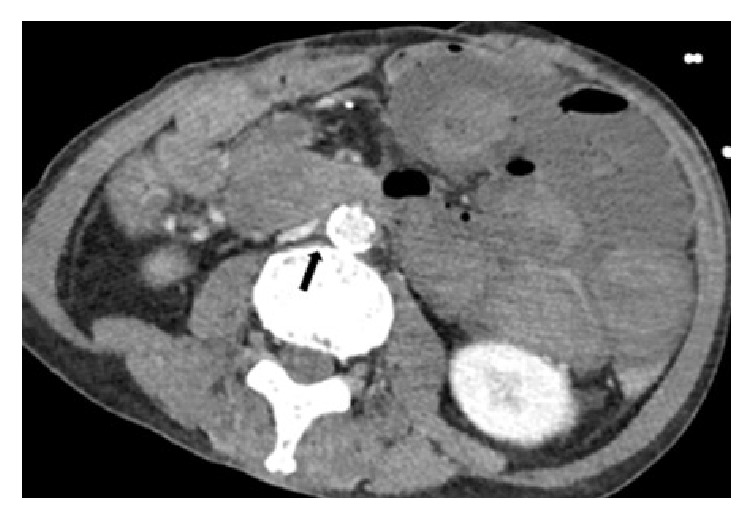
Contrast-enhanced computed tomography image on admission. A massive hematoma is visible in the small intestine, with mucosal thickening of the duodenal wall, duodenal stenosis at the level of the third and fourth portions of the duodenum, and soft-tissue density (arrow) with fat stranding between the duodenum and aorta.
